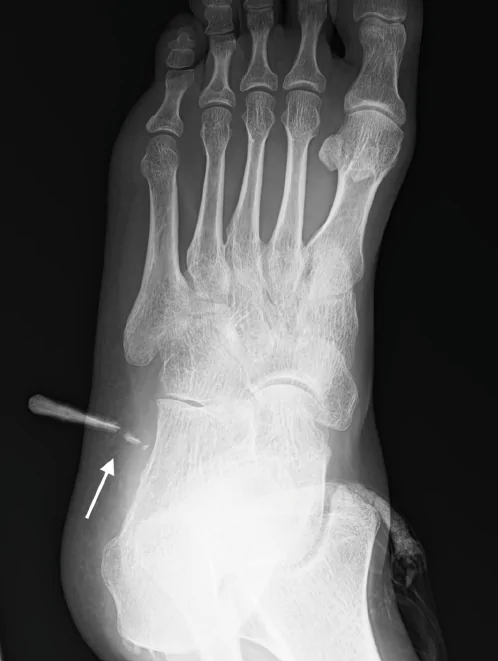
Plain radiograph of the left foot demonstrating a retained stingray barb fragmented into three pieces within the soft tissue.
